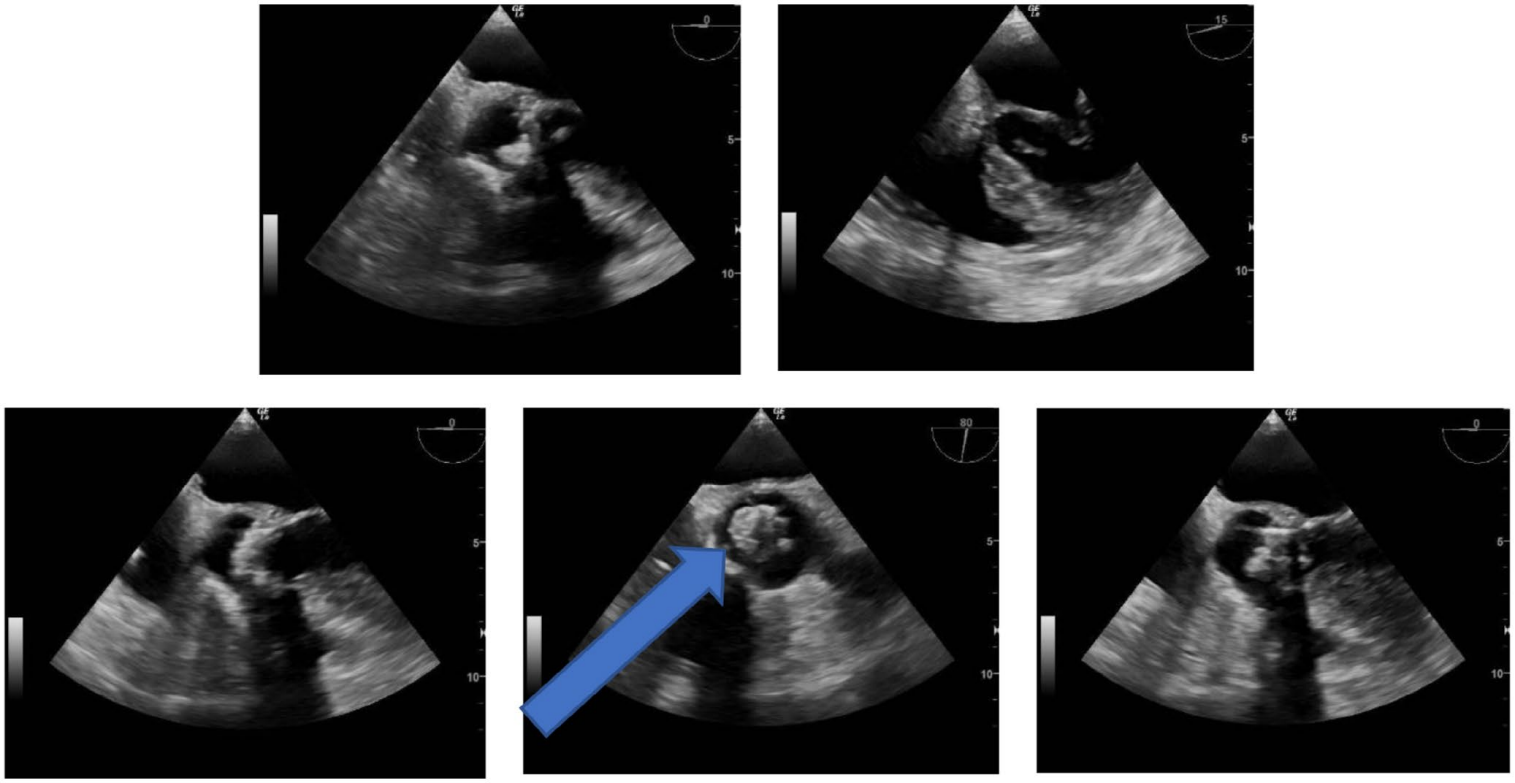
Transesophageal echocardiography showed a thickened biologic aortic valve with probable calcification of the cusps on its tip. The presence of a mobile hyper‐echoic image on a leaflet in favor of vegetations (pointed by the arrow) also is to be noted.
